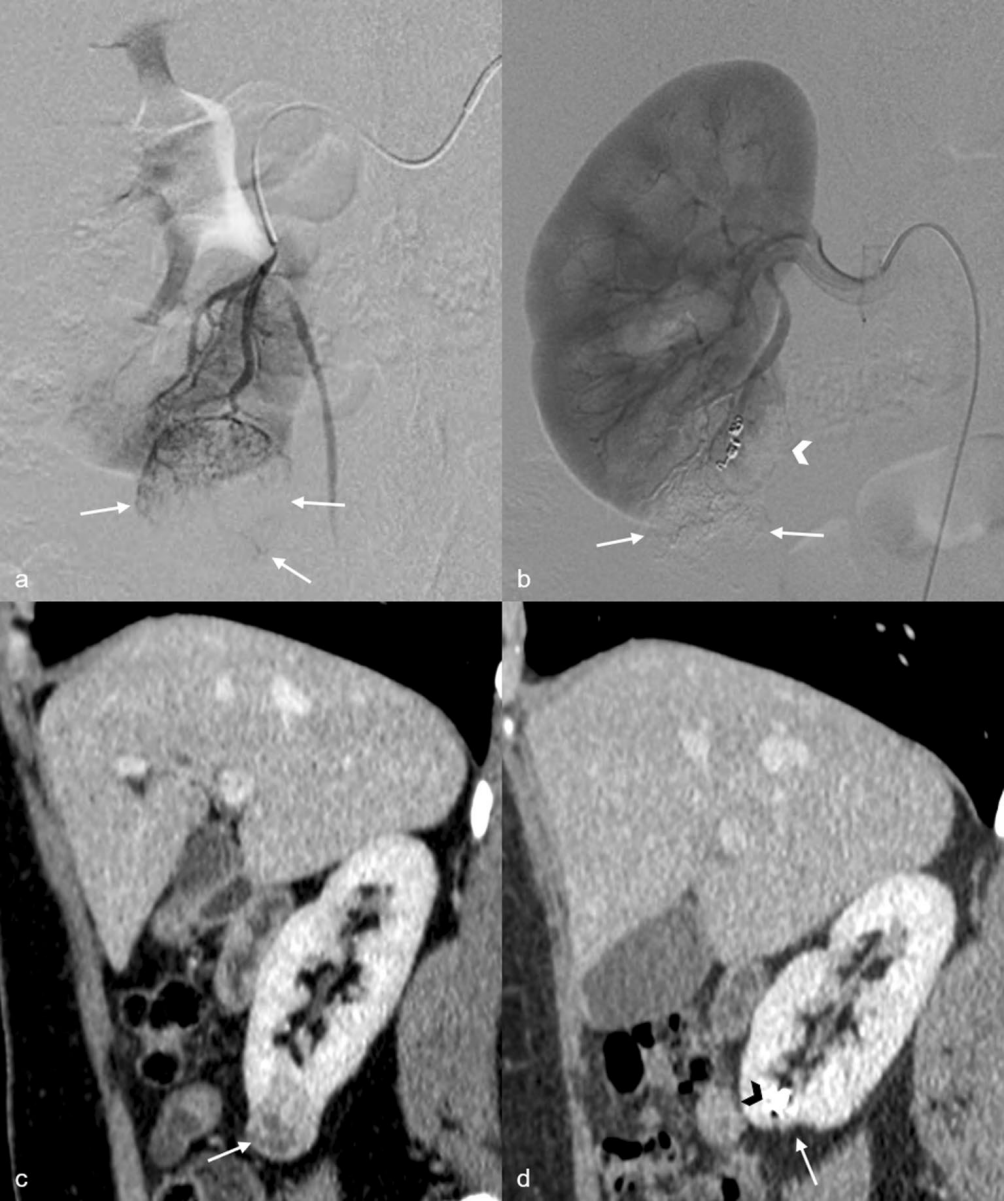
44-year-old woman operated on for right kidney renal clear cell carcinoma (pT1a, complete resection). (a) Selective arteriography shows a tumoral blush at the lower pole of right kidney (arrows). (b) After embolization, the renal arteriography shows complete tumoral devascularization (arrows) with minimal defect (arrowhead) of the peri-tumoral renal parenchyma. (c) Pre-operative CT scan shows the lower polar tumor (arrowhead). (d) Sagittal sections of a tubular time CT scan 6 months post-operatively show the operating area with minimal cortical thinning (arrow) and hyperdense embolization material (arrowhead).
